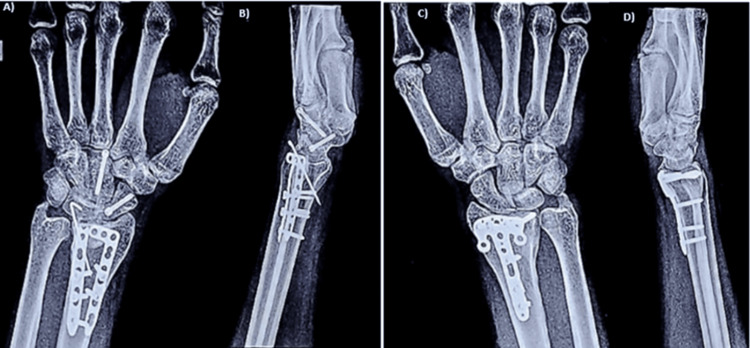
Postoperative X-rays A) Postoperative X-ray of the right wrist in AP view. B) Postoperative X-ray of the right wrist in lateral view. C) Postoperative X-ray of the left wrist in AP view. D) Postoperative X-ray of the left wrist in lateral view
